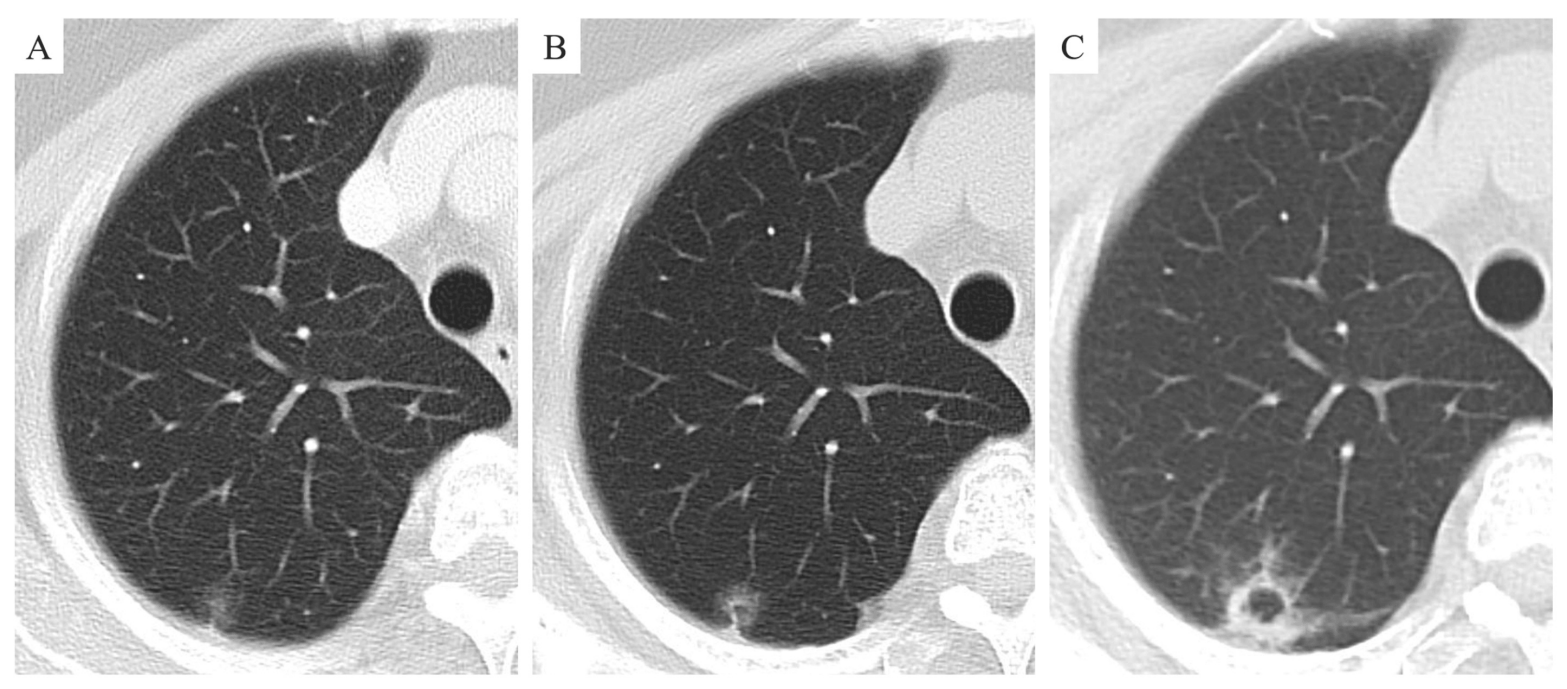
Computed tomography scans of the lung nodule over two years. Serial CT scans obtained at one-year intervals. A ground-glass nodule was initially detected (A), which gradually increased in size and density (A–C). By the end of follow-up, the nodule measured 23 mm and contained an internal cavity.
